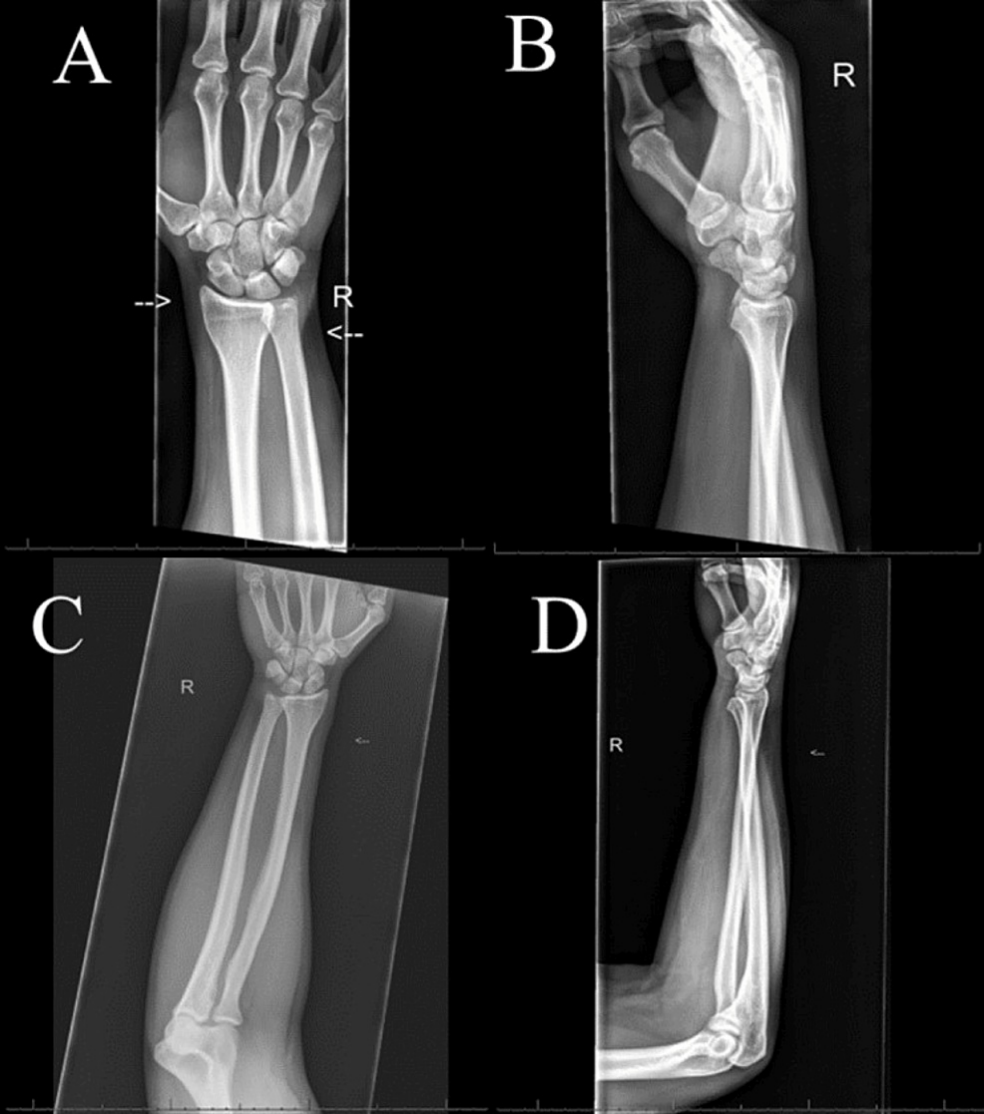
Anterior/posterior and lateral radiographs of the right wrist and forearm. (A and B) Anterior/posterior and lateral radiograph views of the right wrist, respectively, prior to reduction. (C and D) Anterior/posterior and lateral radiograph views of the right forearm, respectively, prior to reduction. These images were initially interpreted as normal but later addended “on the frontal view, there is overlapping of the DRUJ on AP view but looks normal on lateral view, which may be due to suboptimal positioning or DRUJ is unstable and partially subluxed/(dislocated) depending on positioning.”
